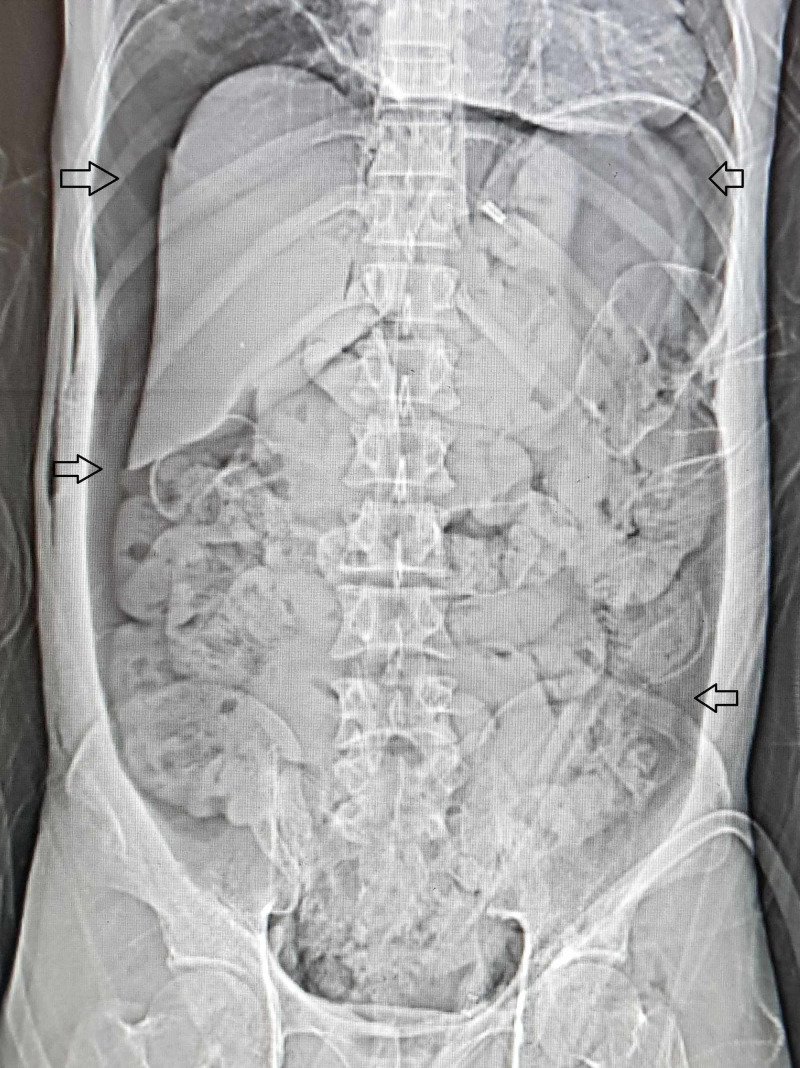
Abdominal radiograph showing the extensive pneumoperitoneum with outlining of the liver, spleen and the small intestine (arrows).
